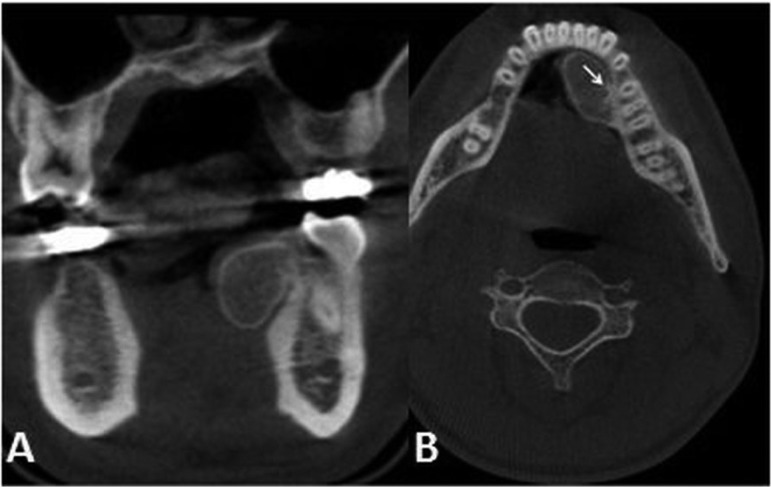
Cone beam computed tomography scan shows the intraosseous cavernous hemangioma. No signs of destruction of the adjacent tissue are present. A) Coronal section showing the bone mass originated from left lingual mandibular region. B) Axial section; the formation of reactive spicula produced by the lesion was indicated by the arrow.
